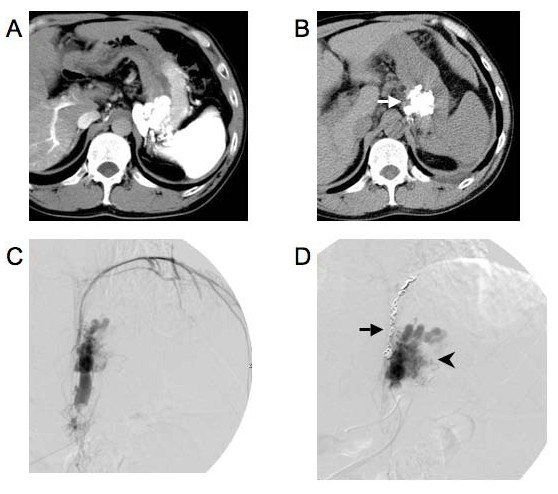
B-RTO performed on a 49-year-old man with alcohol-related liver cirrhosis. A CT scan obtained during arterioportography showed gastric fundal varices. B. Bleeding of the gastric varices occurred before the scheduled B-RTO procedure, and endoscopic injection sclerotherapy (EIS) using cyanoacrylate was performed as an emergency procedure to control the bleeding. A plain CT scan obtained after EIS showed that the sclerosing agent was almost uniformly distributed throughout the network of gastric varices (arrow), except in the gastrorenal shunt. The scheduled B-RTO was cancelled because the bleeding of the gastric varices ceased after the emergency EIS. C. The patient had a history of repeated gastric variceal bleeding and was referred to our department for a B-RTO procedure 4 years after he had received EIS for the first time. The venogram revealed a gastrorenal shunt; however, the gastric varices could not be clearly visualized because the contrast medium had leaked into systemic circulation. D. A retrograde venogram obtained after coil embolization of the inferior phrenic vein (arrow) clearly showed the recurrence of gastric varices (arrowhead). The gastric varices were completely thrombosed after the first B-RTO procedure, as determined by CT during follow-up.
